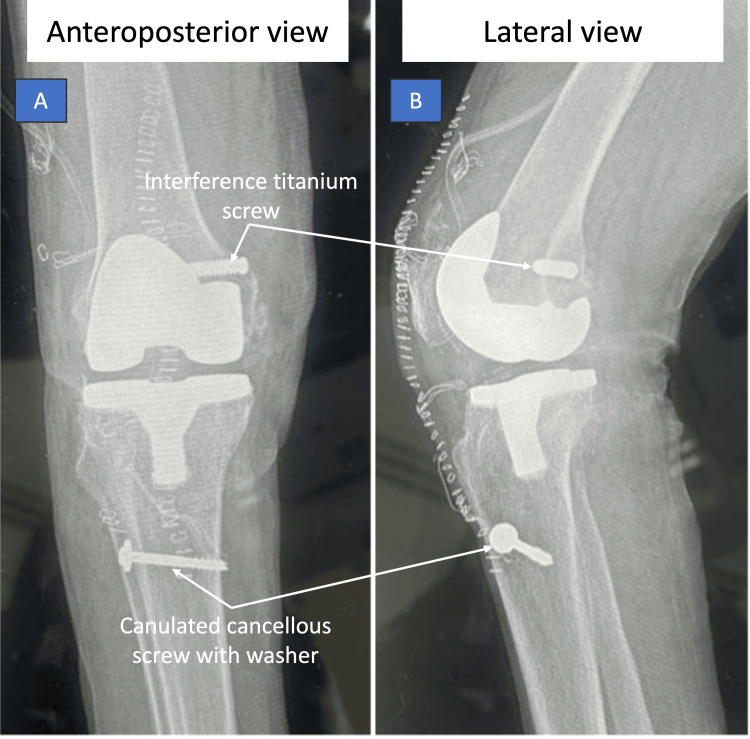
Postoperative radiographs following total knee arthroplasty with ligament reconstruction. (A) Anteroposterior view demonstrating a well-aligned knee prosthesis with a femoral interference titanium screw used for medial collateral ligament fixation. A cannulated cancellous screw with washer is noted on the tibial side securing the posterior oblique ligament graft. (B) Lateral view confirming appropriate positioning of the prosthetic components and fixation hardware, including the femoral interference screw and tibial cannulated cancellous screw with washer.
